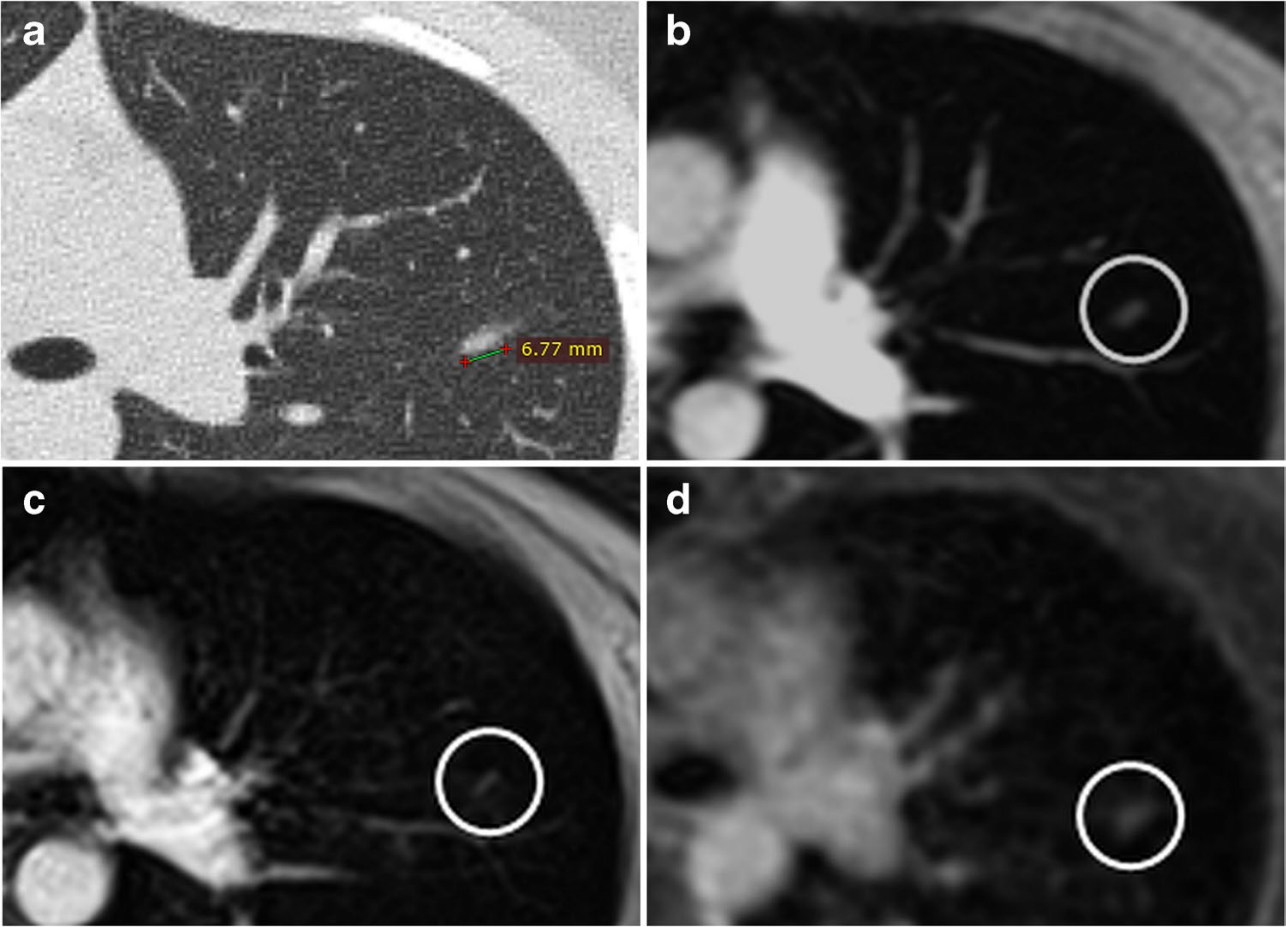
A 10-year-old female patient with rhabdomyosarcoma. An oval-shaped pulmonary nodule with a maximum axial diameter of 7 mm (axial average diameter of 5 mm) was detected on chest CT in the left upper lobe (a). The nodule was not detected with the incoherent GRE sequence (b) and the volume interpolated GRE sequence (c) in the initial blinded session, as the nodule was misinterpreted as part of a vessel due to its linear appearance (circles); furthermore, the lesion was only shown in one image in both GRE sequences due to the slice thicknesses of 4 mm. The oval-shaped nodule (circle) was detected in the UTE image (d) in the initial evaluation session; the lesion was additionally seen in 3 images because of the slice thickness of 1.2 mm of the UTE sequence
